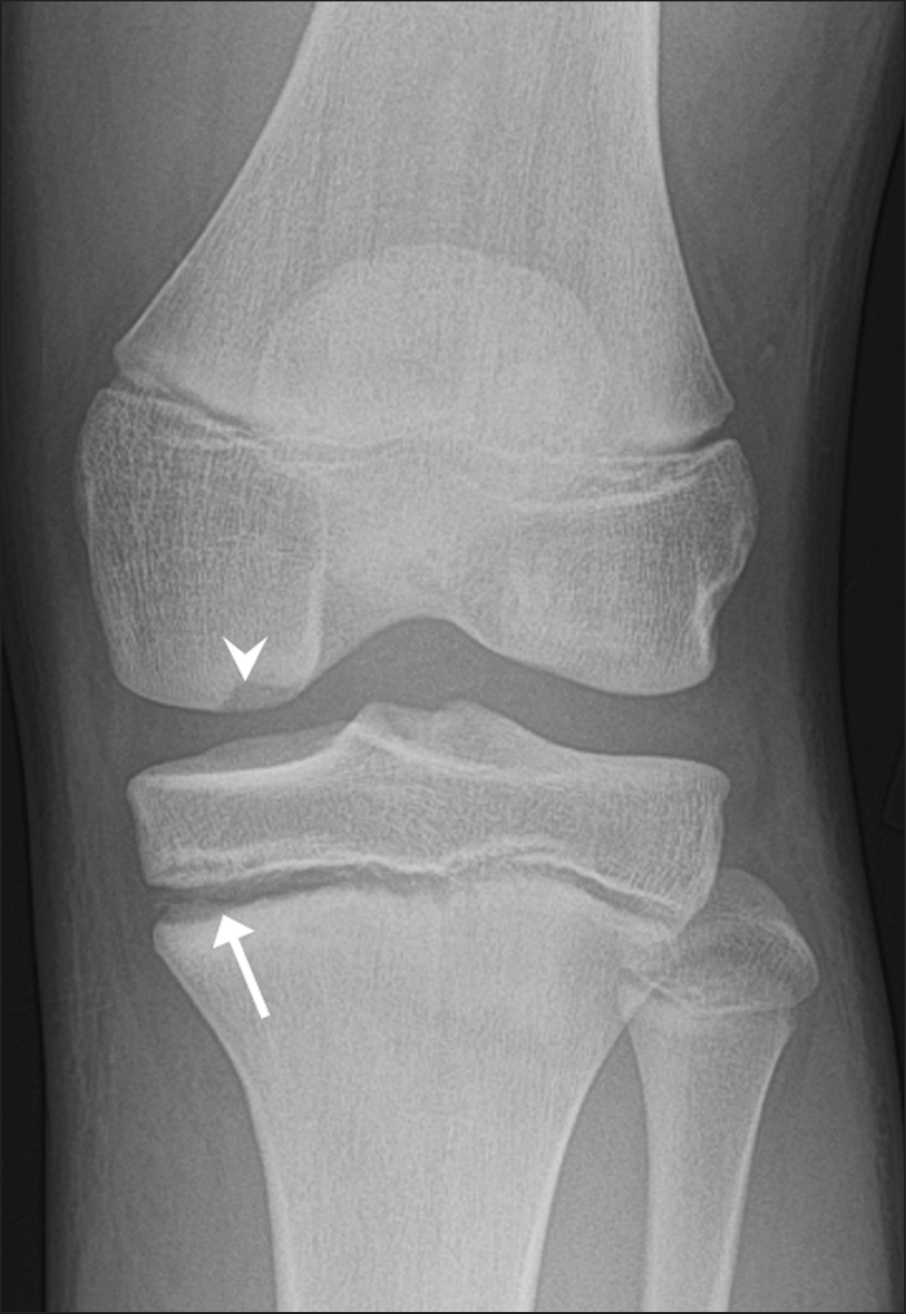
MRI of the left knee was obtained because of persistent widening of the proximal tibia physis. Proton density and fat suppressed proton density sequences confirmed the Salter-Harris I fracture of the medial proximal tibial physis with mild widening and a well-defined band of low signal intensity on all sequences in the medial part of the growth plate (*Figure 2A and 2B, long arrow*) representing trapped periosteum that has displaced from the medial tibial metaphysis (*dashed arrow*). Also note the slight periosteal stripping and tear along the lateral tibial metaphysis without displacement (*Figure 2B, arrowhead*). Additionally, there was a moderate grade injury of the medial collateral ligament and pes anserine, which are partially shown (*Figure 2B, dotted arrow and short arrow*), and extensive soft tissue oedema.
